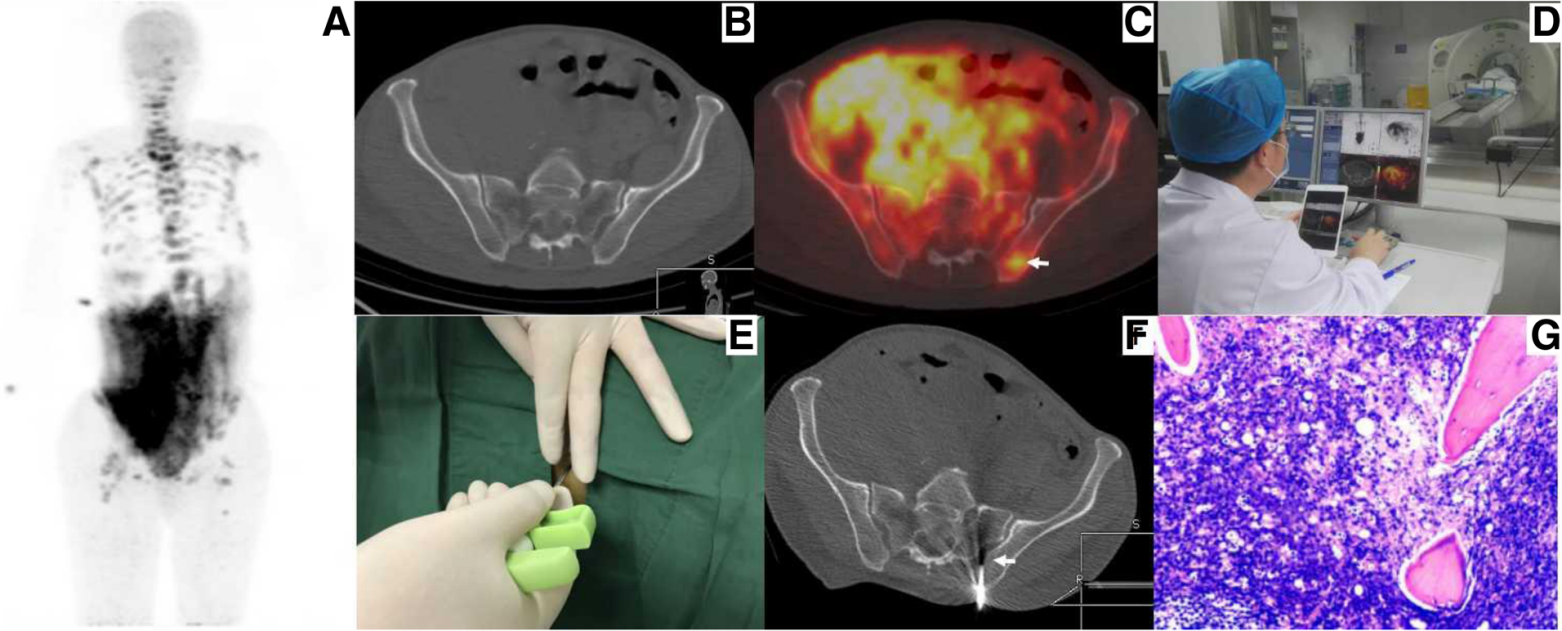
PET/CT-guided targeted bone marrow biopsy procedure. a-c
